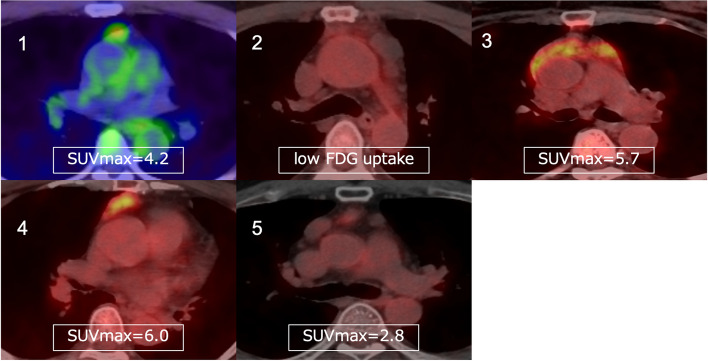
PET-CT scan findings Faint accumulation was only observed in case 2. Meanwhile, evident accumulation was noted in the other cases
